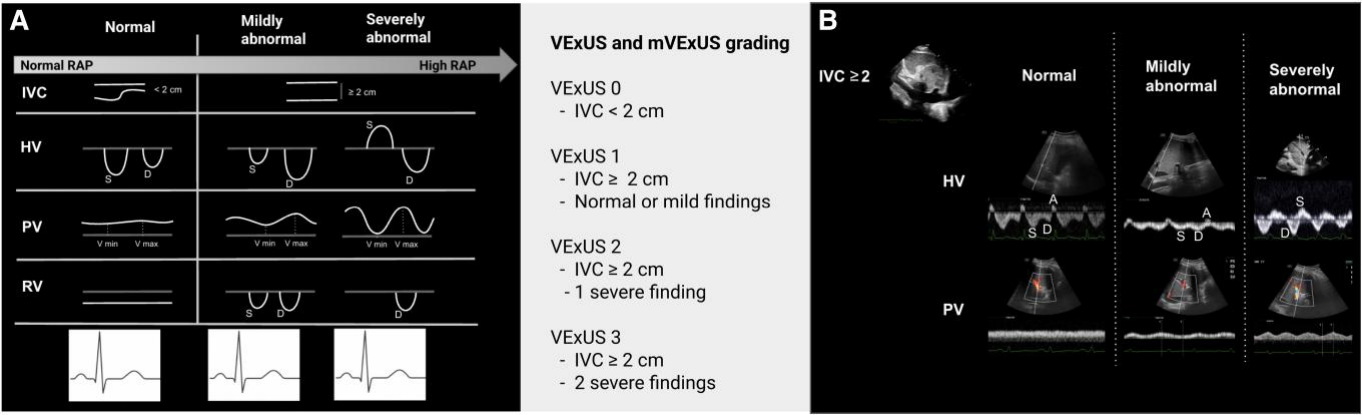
VExUS grading system and modified protocol. (*A*) Visual summary of the four VExUS grades, integrating IVC diameter and venous Doppler patterns from the HV, PV, and RV to stratify the severity of systemic venous congestion. (*B*) Modified VExUS (mVExUS) protocol, which excludes the renal vein. Flow patterns from HV and PV are categorized as normal, mildly abnormal, or severely abnormal to determine the final grade.
